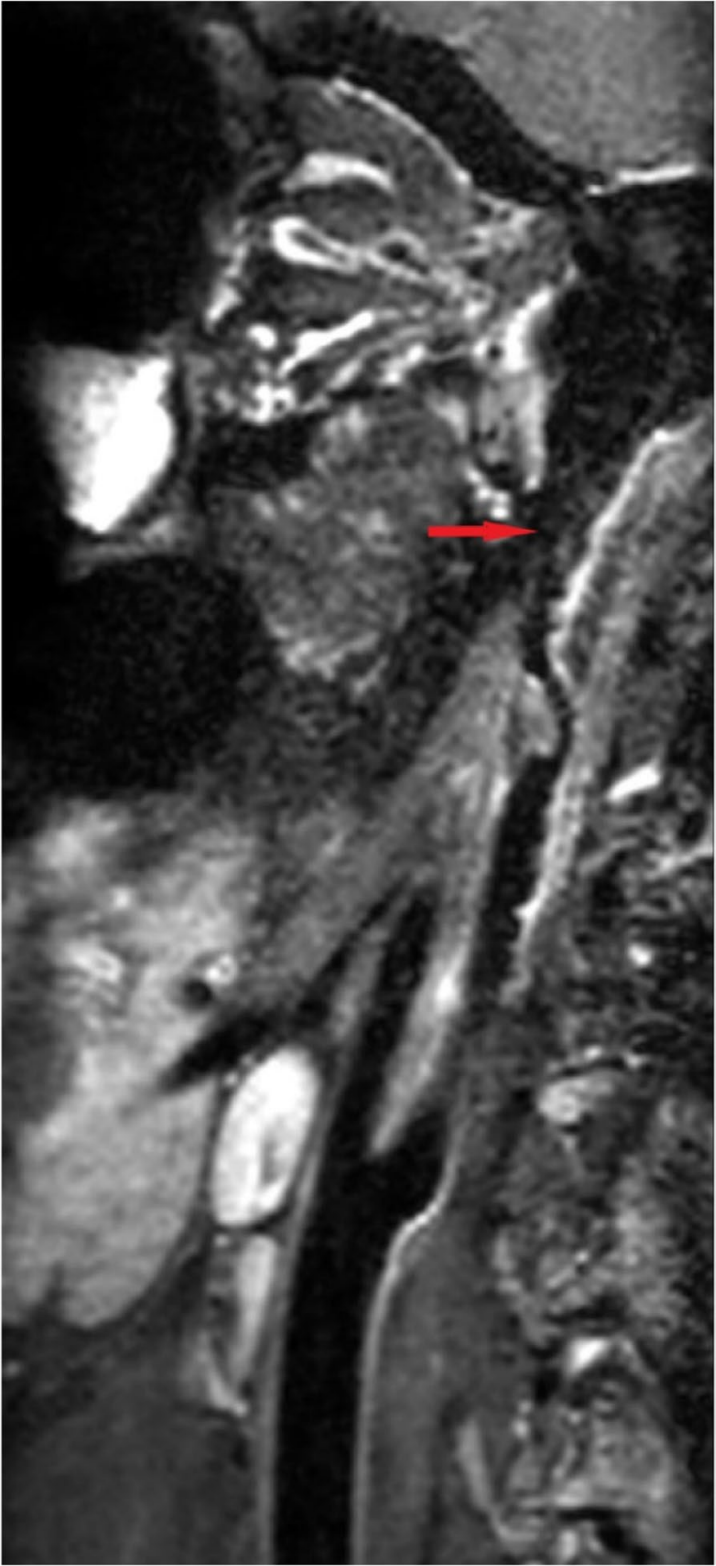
High-resolution magnetic resonance imaging shows enhanced signal on the surface of the atherosclerotic plaque, representing thrombosis, which has caused moderate to severe stenosis
